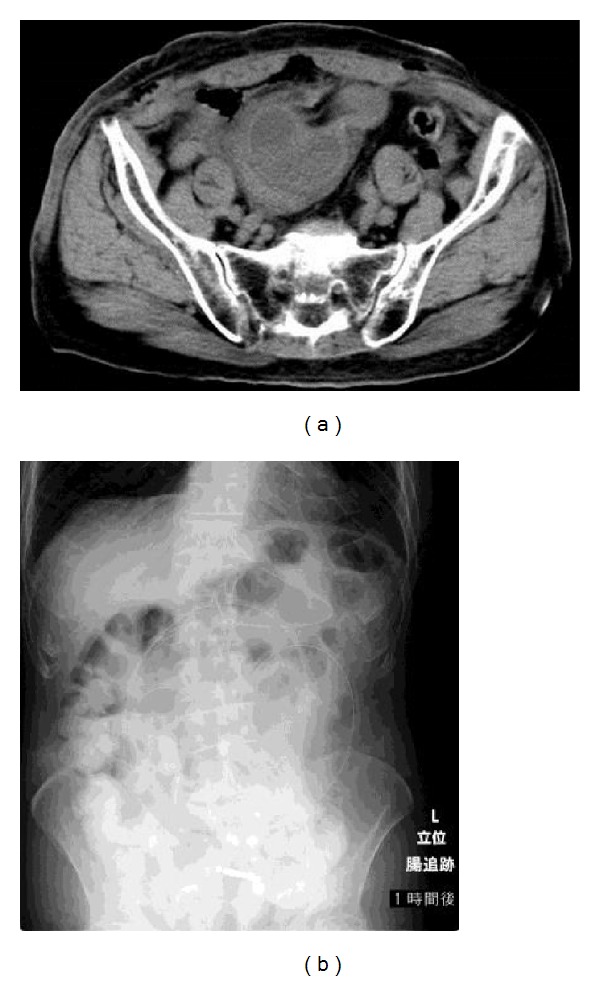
(a) A cyst-like bowel loop is visible in the pelvic cavity. Bowel obstruction is not complete, and localized adhesion of the small bowel is thought to be due to repeated prolapse and incarceration over many years. (b) Abdominal X-ray image 1 hour after injection of contrast agent from the ileus tube. Contrast agent has reached the colon, the small bowel is not dilated, and bowel obstruction has improved.
